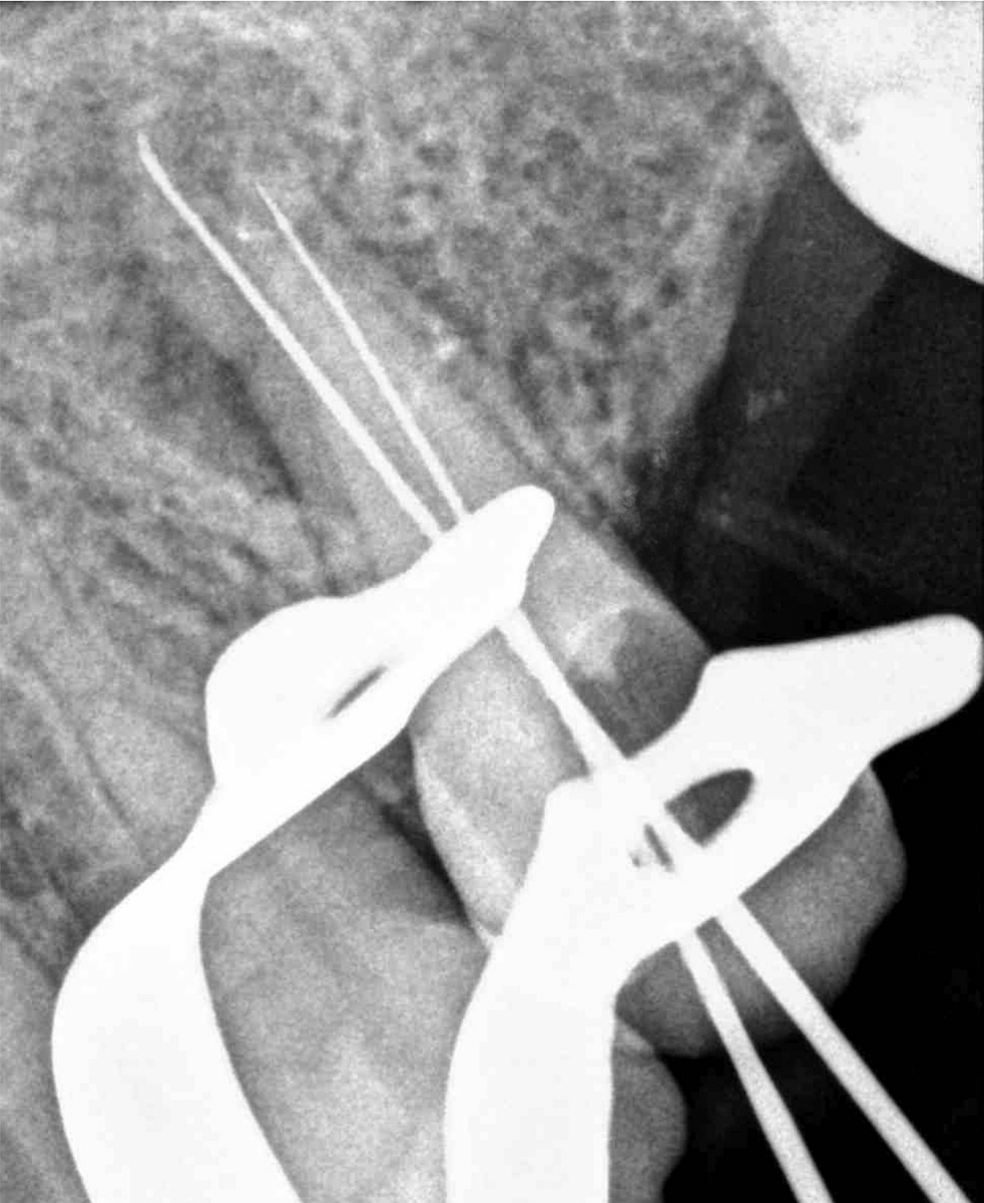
Working length was determined using an apex locator and confirmed with an intraoral periapical radiograph.
